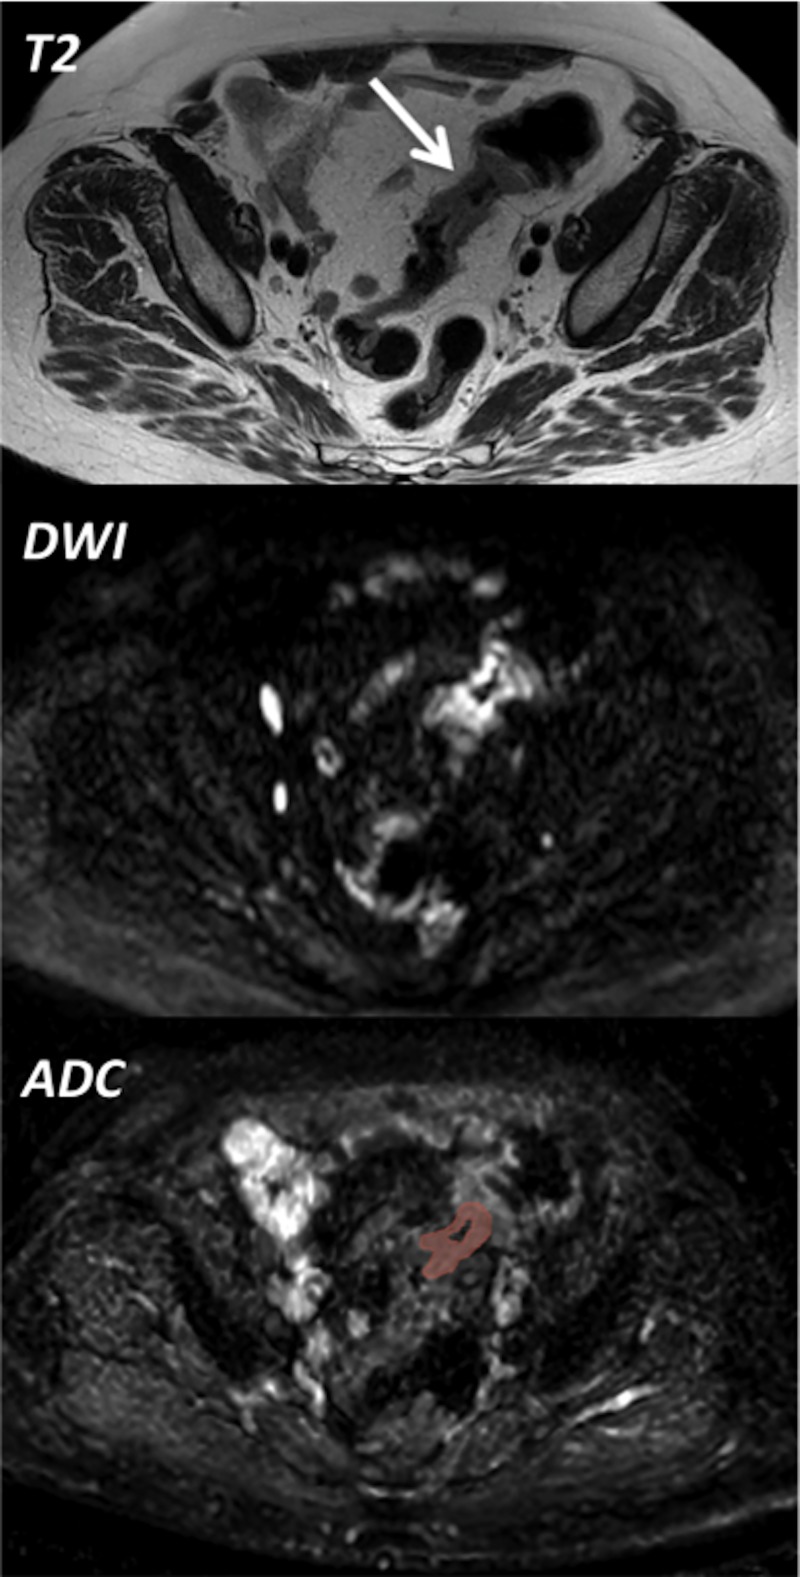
Example of image processing. The T2 weighted MRI sequence (top picture) is showing the tumor in the sigmoid colon (arrow). The middle picture is the corresponding b1000 weighted DWI (Diffusion Weighted Imaging) sequence showing a high signal intensity within the tumor (consistent with malignancy). Bottom picture shows an example of tumor delineation on the corresponding ADC (Apparent Diffusion Coefficient) as performed to obtain the mean ADC-value of the whole tumor. An abdominal radiologist with 13 years experience in reading abdominal MRI and a radiology resident delineated each tumor in consensus directly on the ADC map whilst referring to the T2 weighted images (top) and the DWI sequence (middle) for anatomical reference.
